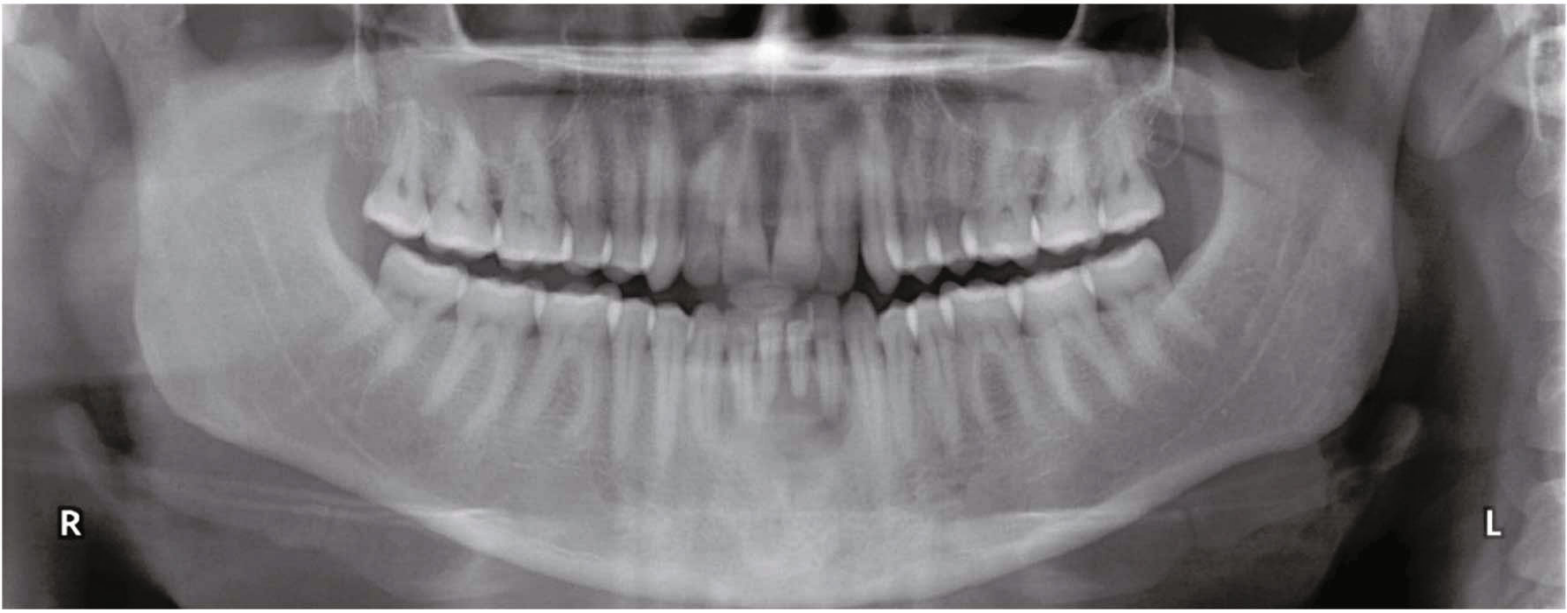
Orthopantomogram showing the mandibular radiolucent area.
